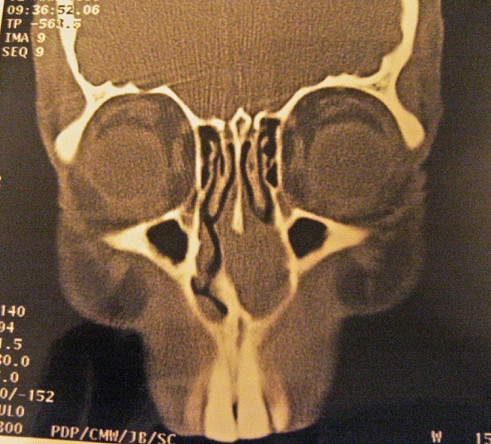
Sinus computed tomography scan (coronal section) showing a 2 × 2.2 × 1.4 cm mass in the left nasal cavity.
